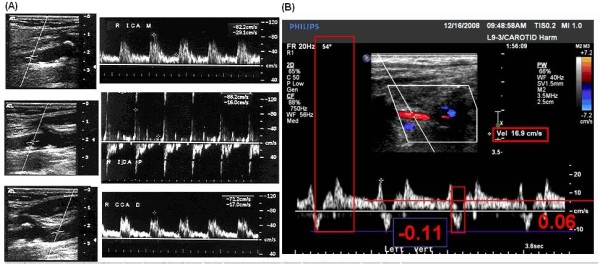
Velocity Reversal and Flow Reversal. A. Lower and Upper Normal waveforms indicate a vessel without stenosis. Middle: Complicated velocities in the region of the bifurcation appear dominated by reversed velocity often called "flow separation". B. Systolic reversal in vertebral arteries is a frequent finding. The examining sonographer marked the EAV as the systolic velocity and did not mark an EDV. The UWURC reader marked the negative systolic peak.
